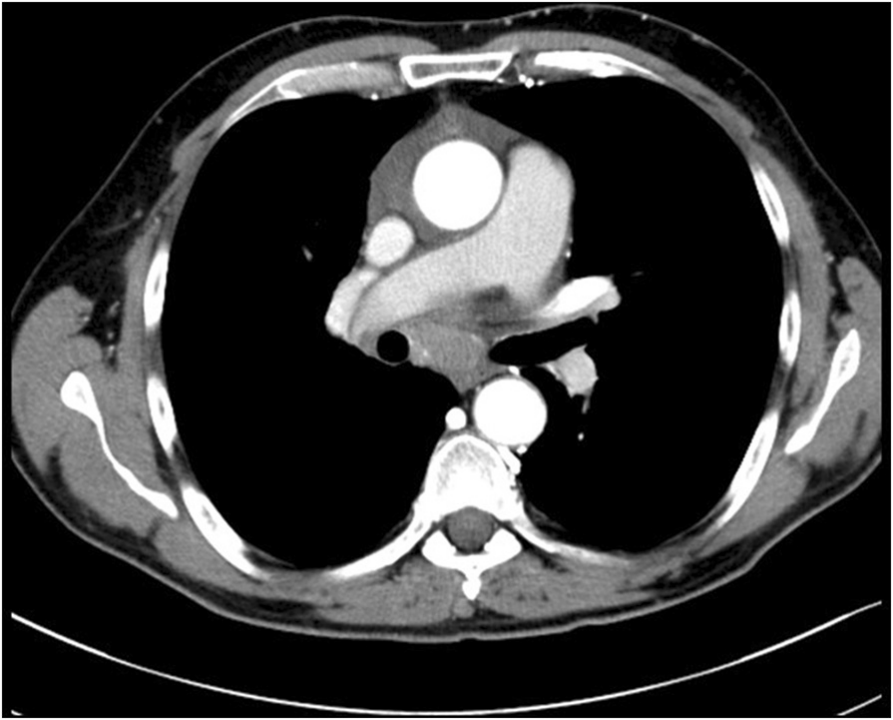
Computed tomography image of subcarinal lymphadenopathy
